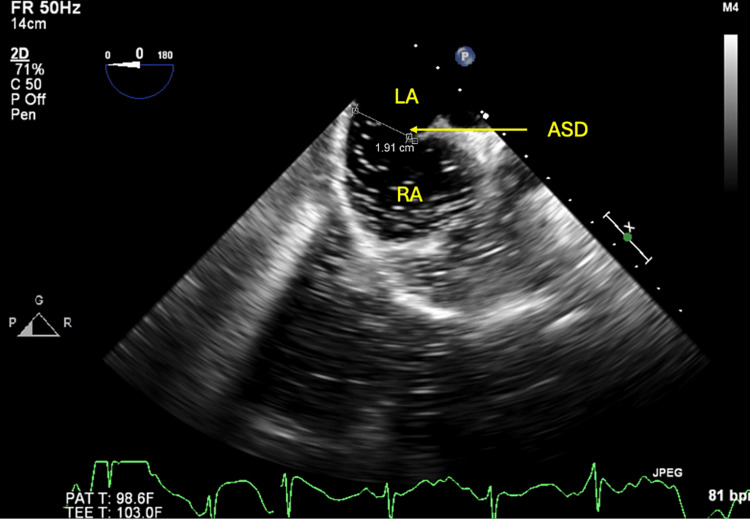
Transesophageal echocardiogram with agitated saline Transesophageal echocardiogram in the bicaval view demonstrates an atrial septal defect measuring approximately 1.91 cm. A left-to-right shunt is demonstrated at the atrial level through the usage of agitated saline contrast. ASD: Atrial septal defect; LA: Left atrium; RA: Right atrium.
